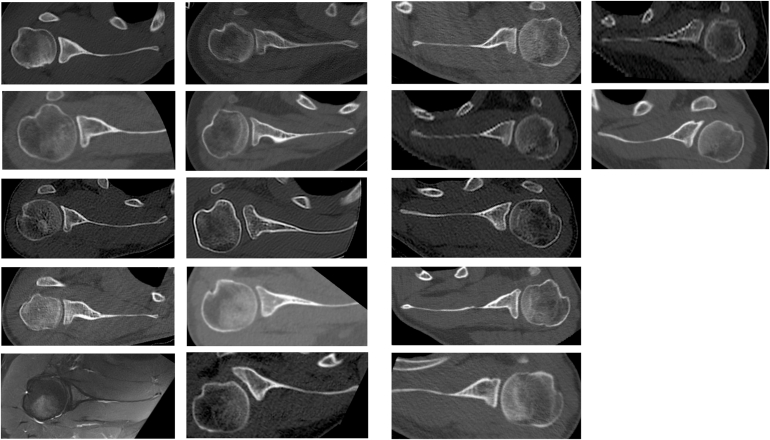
CT/MRI scans of measured affected shoulders. Ten right-side affected presented here, with 9 CT scans and 1 MRI scan, and 7 left-side affected presented with CT scans. *CT*, computed tomography; *MRI*, magnetic resonance imaging.
